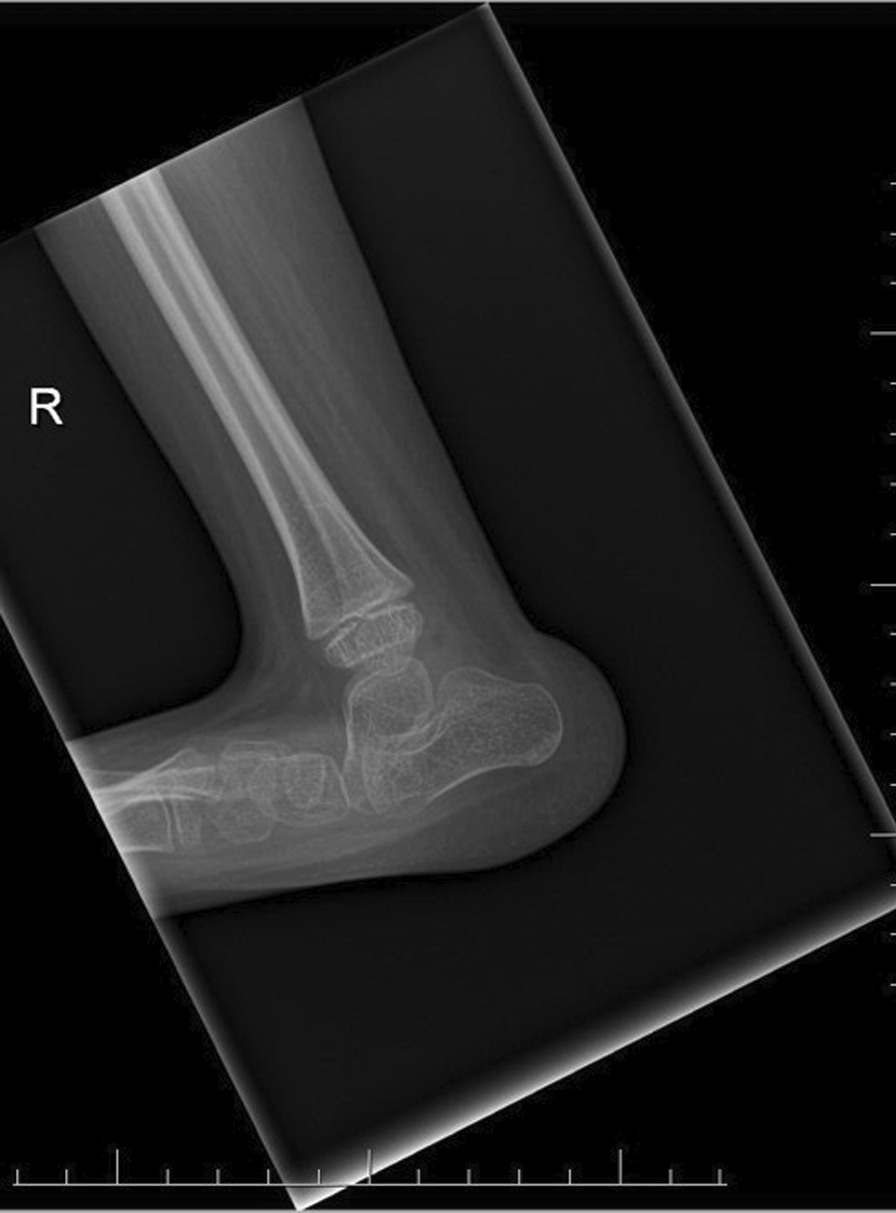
Case 2 AP and L X-ray before surgery
